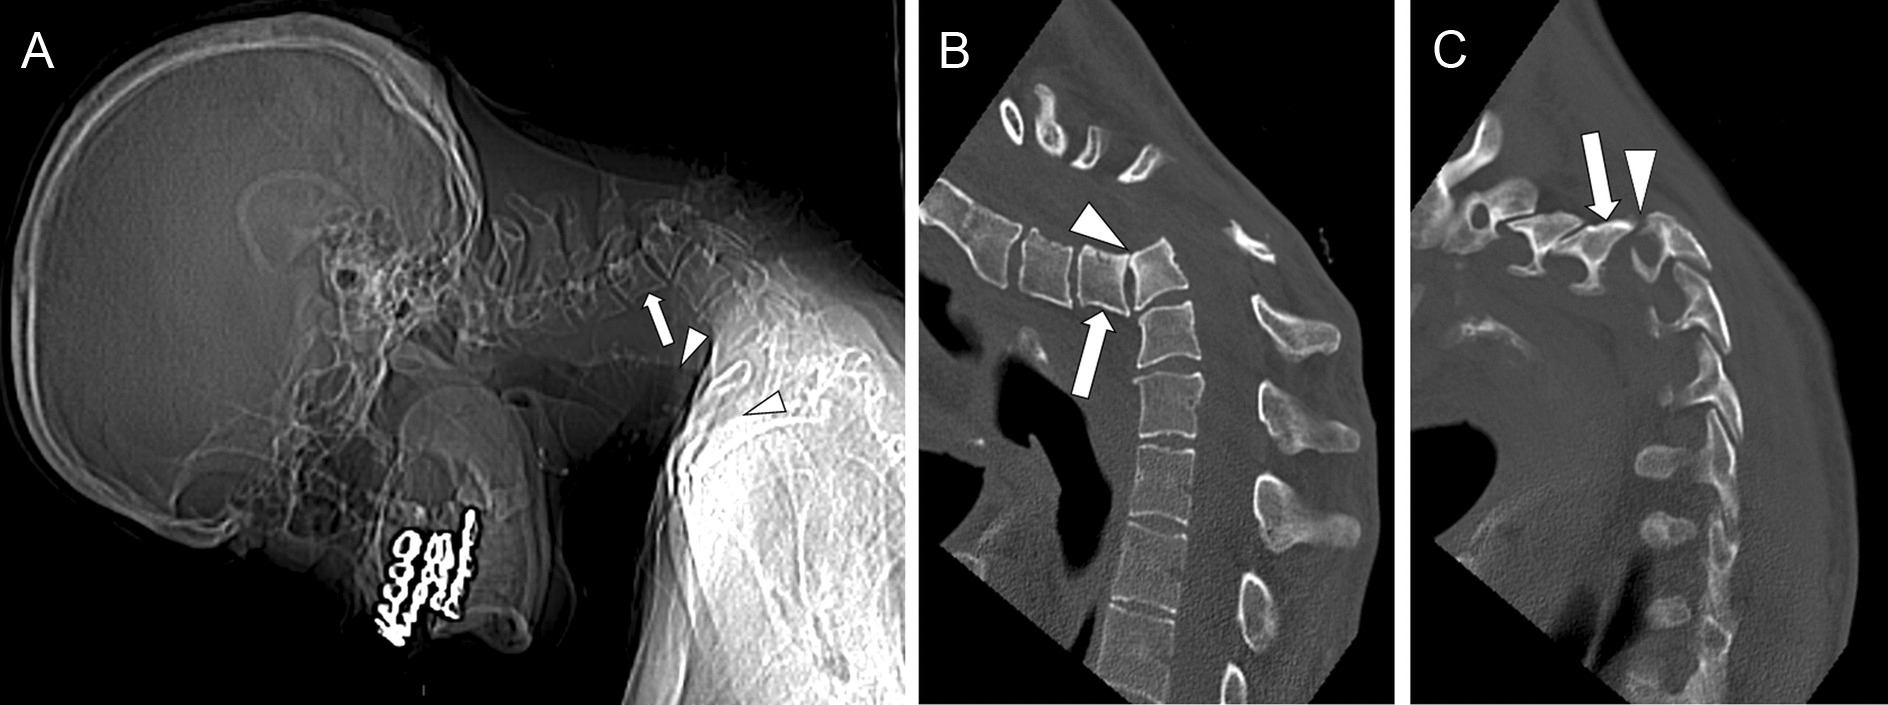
Initial computed tomography: scout view (A) showing the C4–C5 complete dislocation with spondyloptosis of C4 (arrow) and severe local kyphosis resulting in flexion of the head on the anterior chest wall with evident sinuosity of the respiratory tract (arrowheads, showing angulation of the trachea). Note the close proximity between the anterior aspect of the mandible and the sternum. Reformatted sagittal images on the midline (B) and at the level of the right facet joints (C) show complete dislocation at the C4–C5 level, with spondyloptosis of the C4 vertebral body anteriorly to C5 (arrow B) and complete anterior translation of the inferior C4 facet joint “locked” at the anterior aspect of the superior C5 facet (arrow in C). Sclerosis of the adjacent vertebral angles (arrowhead in B) and periarticular calcifications posteriorly (arrowhead in C) indicate the dislocation as established for several weeks
